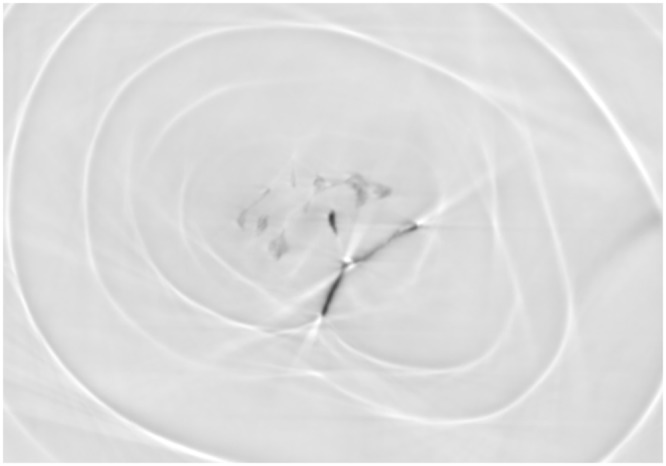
The “moustache” of the cultured freshwater pearl CP-21 revealed by the X-ray PC tomography.
